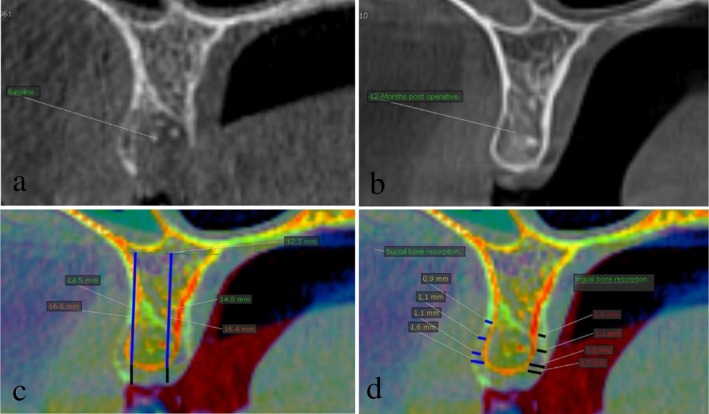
(a) Baseline CBCT scan showing alveolar ridge configuration immediately after the ARP procedure; (b) 1‐year follow‐up CBCT scan demonstrating bone formation at the site of preservation with proper ridge morphology; (c) a coronal view of the superimposed baseline (the black vertical line) and follow‐up (the blue vertical line) scans to demonstrate the vertical dimensional change that has occurred throughout a year of follow‐up; (d) a coronal view of the superimposed baseline and follow‐up scans to demonstrate the buccolingual dimensional change that has occurred throughout a year of follow‐up.
